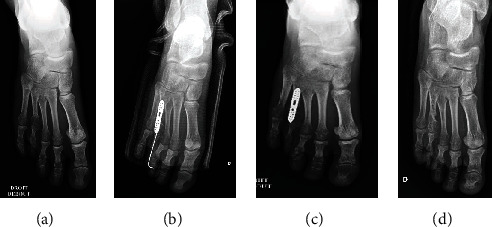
Case 1, a 20 years old female with a brachymetatarsia of the right fourth toe. (a) Preoperative X-ray of the right foot showing the shortness of around 10 mm. (b) Postoperative X-ray of the right foot. (c) Postoperative X-ray after K-wire removal at 1-month follow-up. (d) Postoperative X-ray after plate removal at 1-year follow-up.
